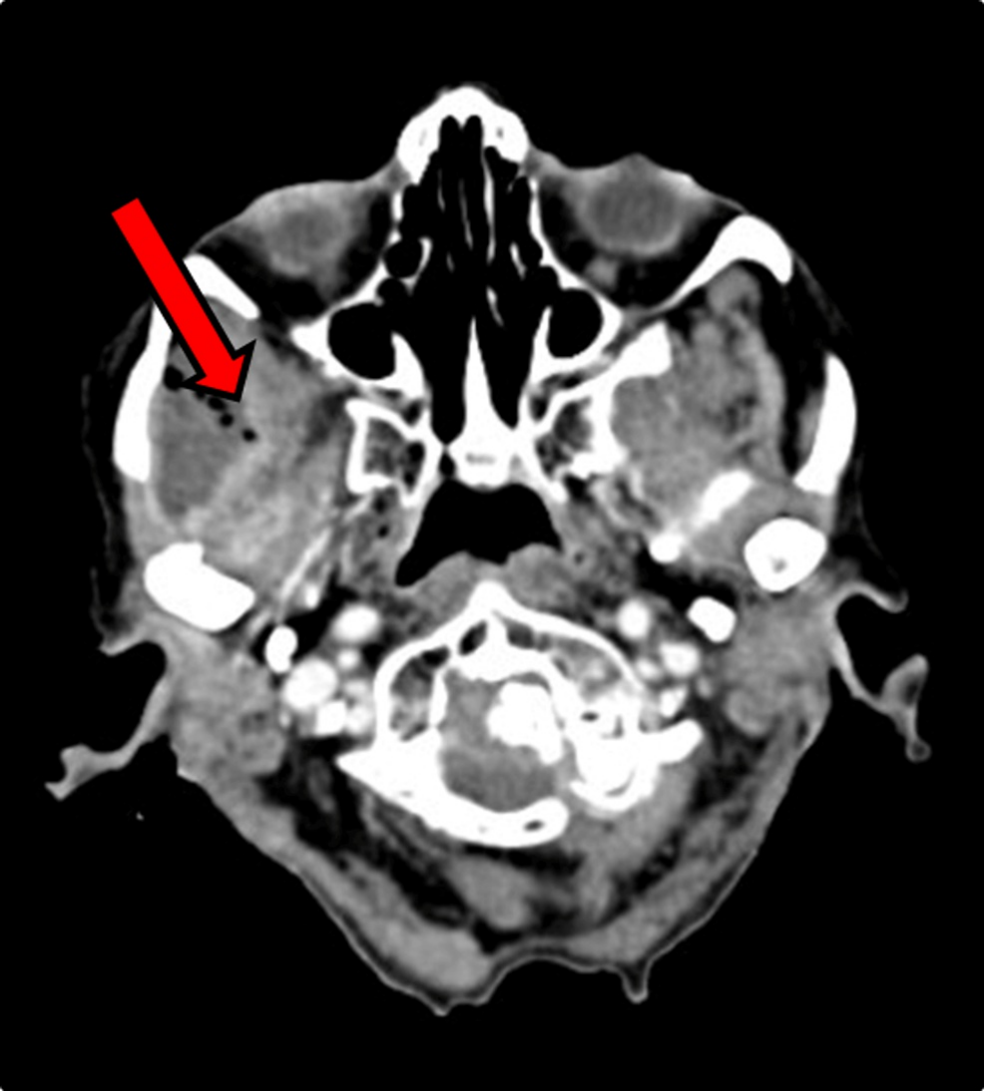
Axial Section of Contrast-Enhanced Computed Tomograph (CECT) Head There is evidence of peripherally enhancing collection with internal enhancing septations within noted over the right frontoparieto temporal regions with multiple air density foci within. The collection approximately measures 9.5 x 2.9 x 12 cm (Axial Plane Topography with Volume Computerized Tomography Contrast (APTVCC)). Inferiorly collection is seen extending into the right infratemporal fossa, masticator, and submandibular spaces. There is involvement of the medial pterygoid and superficial and deep fibers of the temporalis muscle. Masseter muscle also shows heterogeneous enhancement and is mildly bulky as compared to the opposite side.
